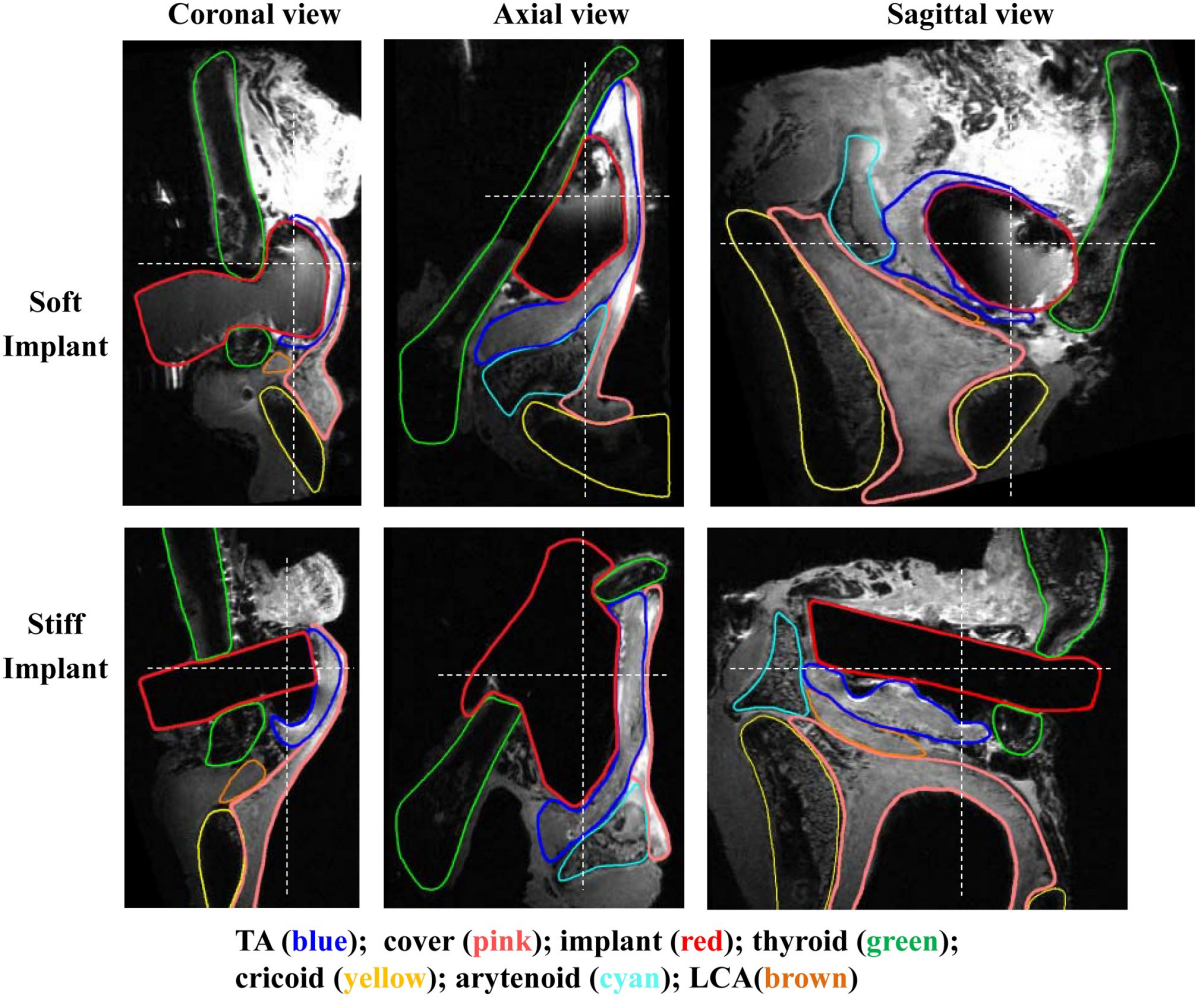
MRI images of larynx L3 (72 years old male) in three views. Top: the left half larynx with insertion of a soft implant. Bottom: the right half with insertion of a stiff implant. The dashed white lines in each view indicate the cut planes from which the other two views were generated.
